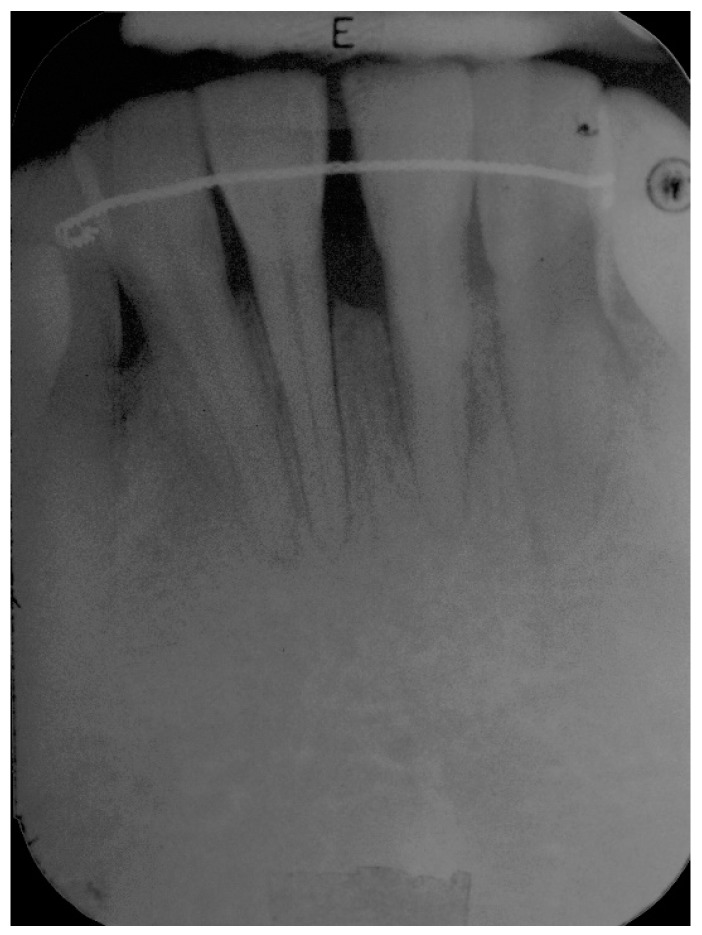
Six-months post-operative radiograph, improved bone support, especially around the central left incisor can already be appreciated.
